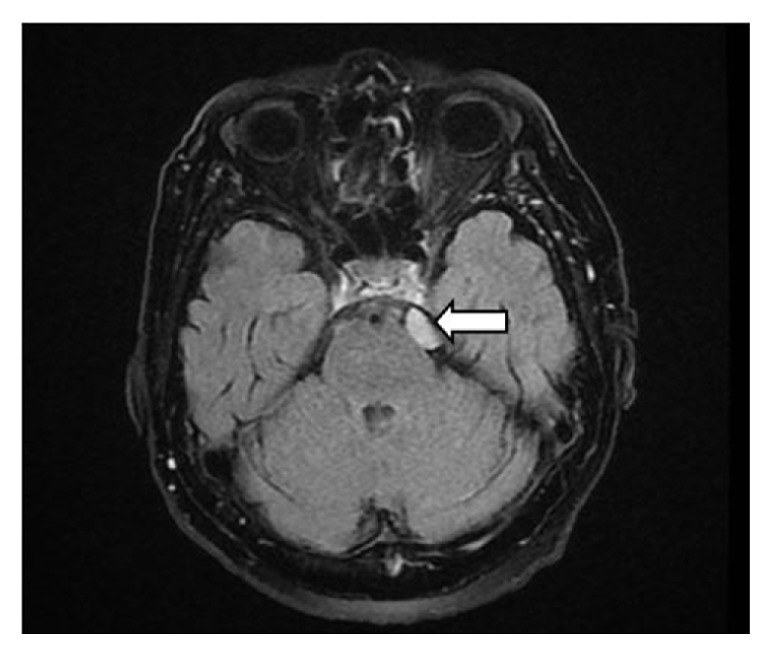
Trigeminal meningioma: cerebral MRI-T2 flair sequence.
